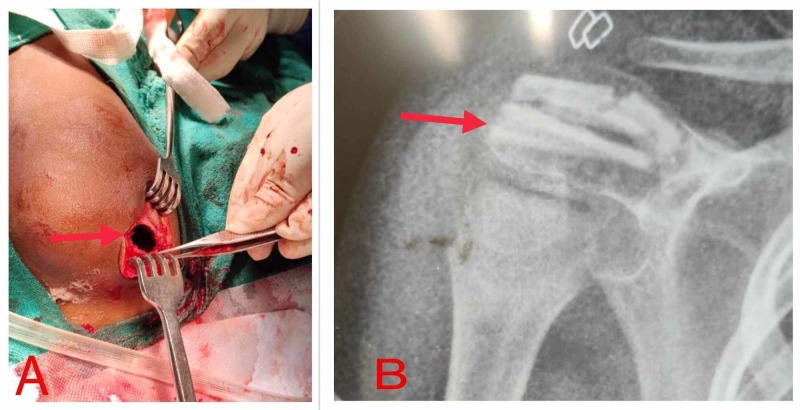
Extended curettage and fibular grafting (A) Posterolateral surgical approach; (B) postoperative X-ray.
